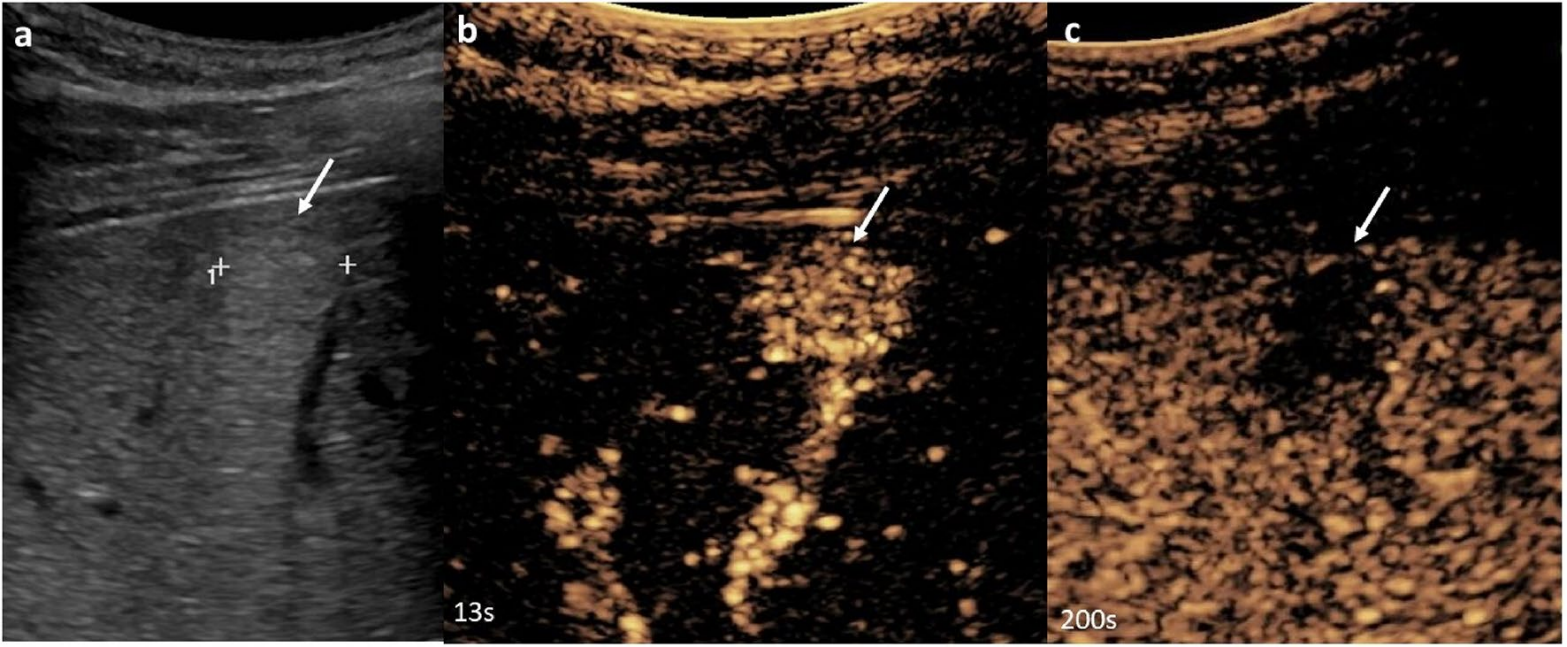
18-year-old male, background of Li Fraumeni syndrome. CEUS of the liver performed to characterize multifocal liver lesions detected on prior imaging. a Grayscale ultrasound showed multiple hyperechoic lesions throughout the liver, with b homogeneous hyperenhancement on arterial phase and washout on c late venous phase, in keeping with malignancy. Colonoscopy showed a sigmoid colonic mass; biopsy showed adenocarcinoma
